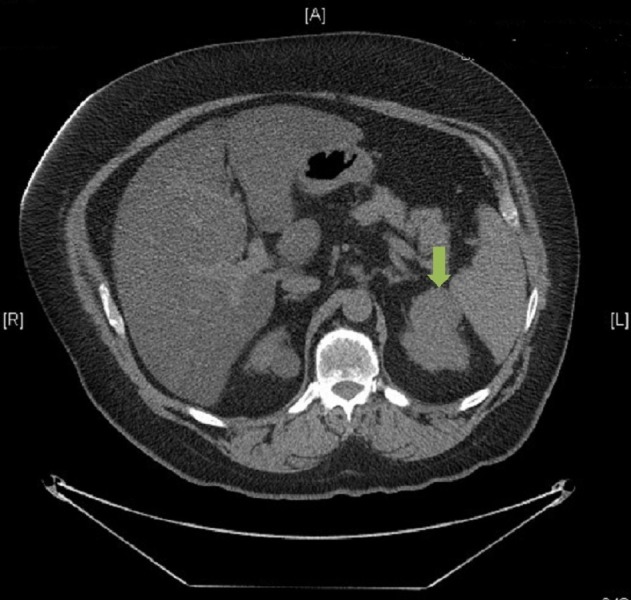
Preoperative CT of the Abdomen Axial slice. Contrast-enhanced image that demonstrates a 4.9 cm isolated clear cell renal cell carcinoma involving the upper pole of the left kidney.
